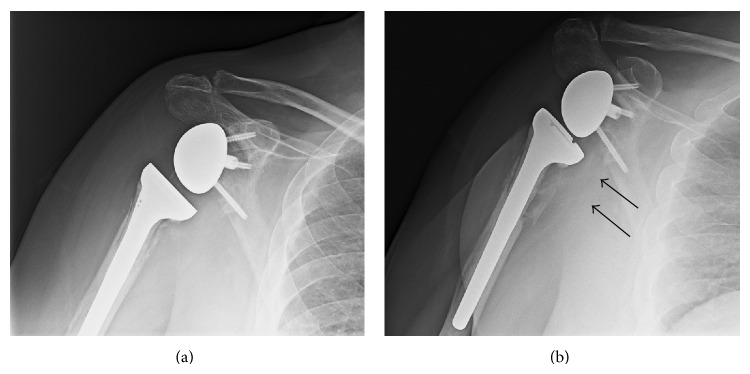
Radiograph showing (a) the reverse shoulder arthroplasty five months after implantation and (b) the reverse shoulder arthroplasty with the enlarged lucencies (arrows) at 18 months after implantation, which is just prior to its removal.
